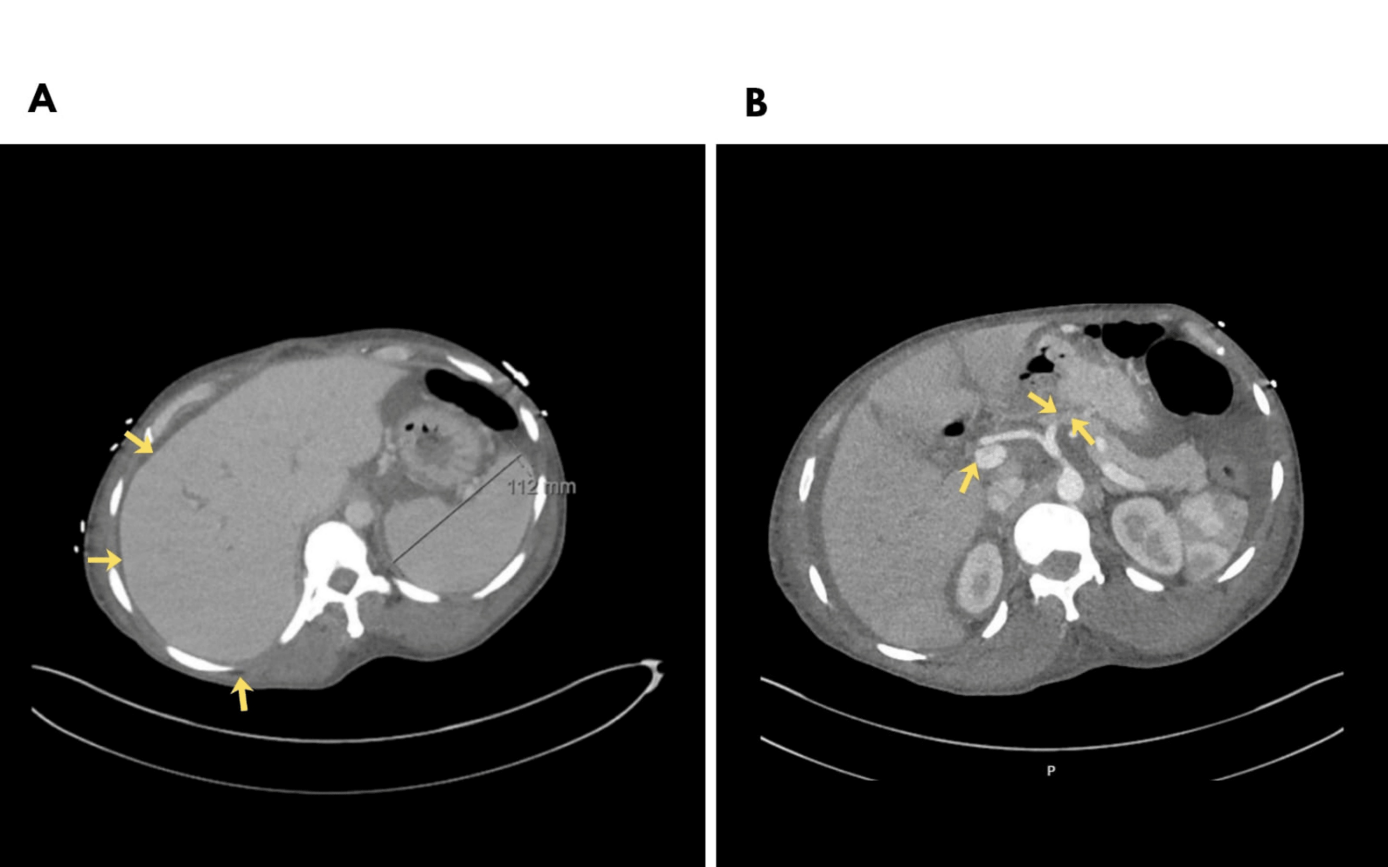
Axial sections of the triple-phase liver CT showing (A) splenomegaly (12.9 cm) with hepatic congestion and (B) thrombus within the hepatic veins CT: computed tomography.
